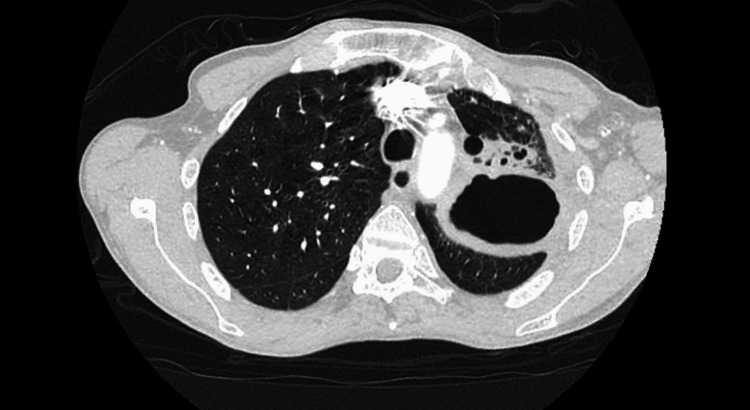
CT of the chest showing 6.8 X 5.1 X 6.7 cm cavitary lesion in the left hilum and left upper lobe
